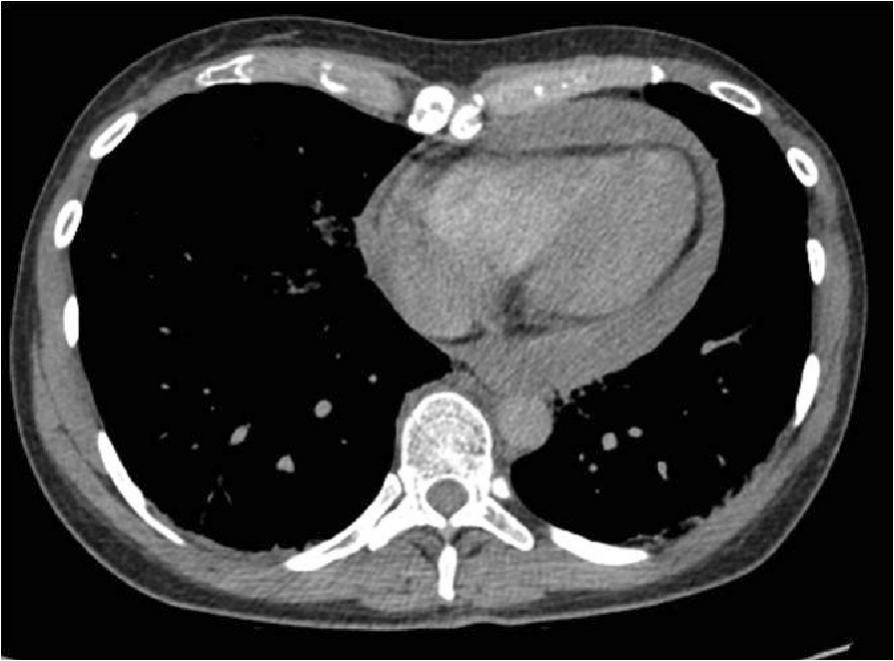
Computed tomography scan showing the three-cm pericardial effusion.
